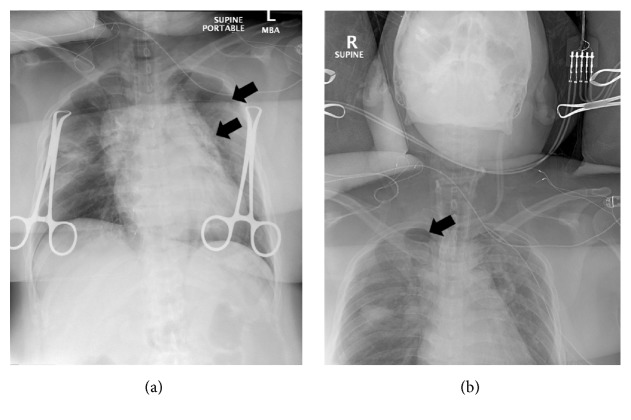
X-rays during surgery showing pneumomediastinum and pneumothorax. First intraoperative chest X-ray revealed a small left-sided pneumothorax and a mild pneumomediastinum ((a) black arrows). Second intraoperative chest X-ray found that the left pneumothorax had decreased in size; however, a new right pneumothorax had developed ((b) black arrows).
